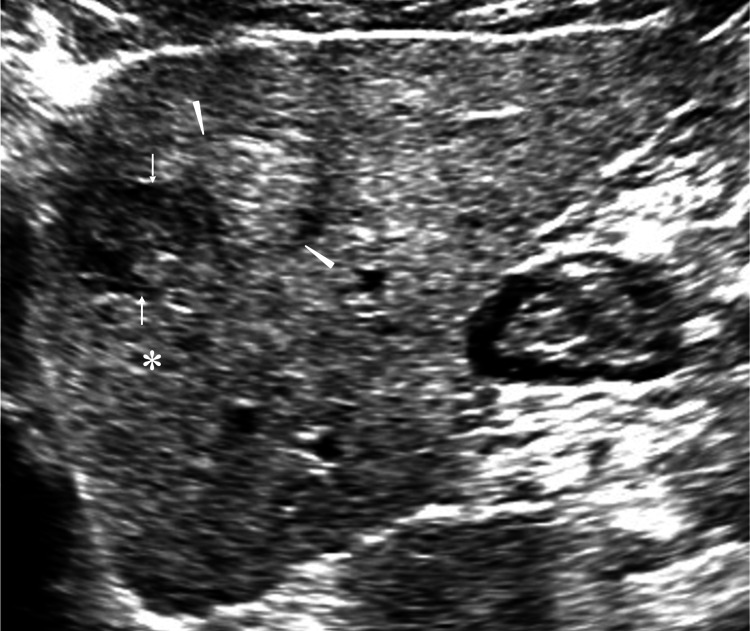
Ultrasound findings Ultrasound showed an oval mass (arrowheads) with predominantly internal high echoes and no enhanced/attenuated posterior echoes (asterisk). Slightly low internal areas (arrows) well corresponded to the edematous fibrous component areas (Figure 4B, asterisk).
